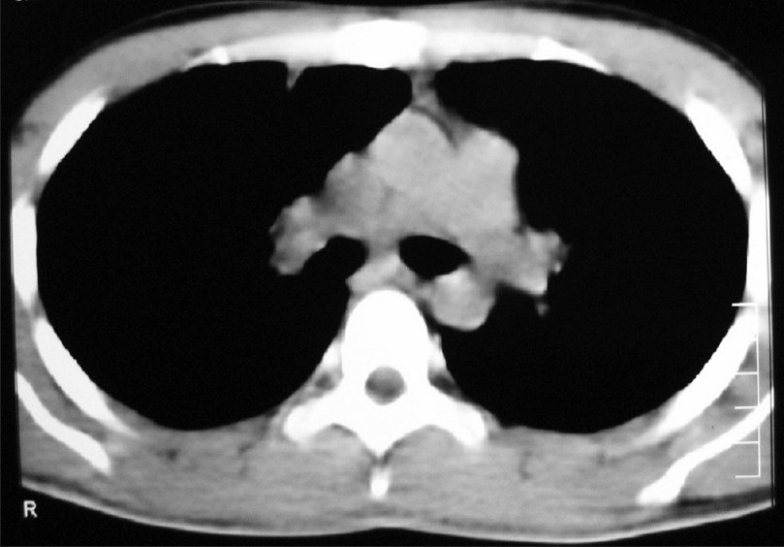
CT chest (2008) shows complete disappearance of the mass after chemo
